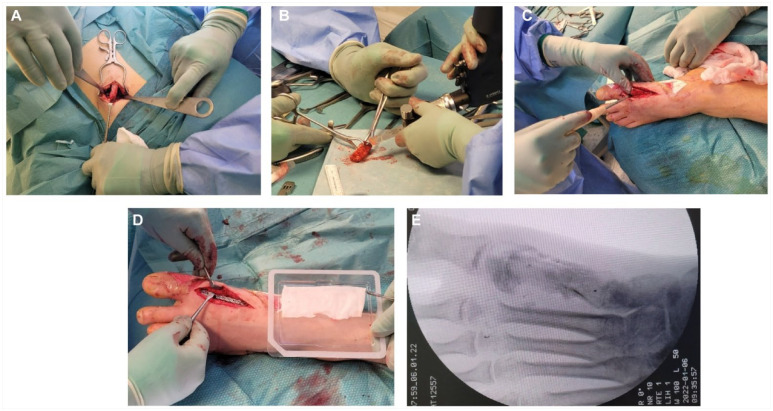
An iliac crest bone graft was harvested from the ipsilateral side (3A), adjusted (3B) and installed into the defect (3C), stabilized with a plate, and finally covered with a sponge soaked with BMP2 (3D). The perioperative radiograph demonstrates the bone graft in the first metatarsal bone prior to plate fixation (3E).
